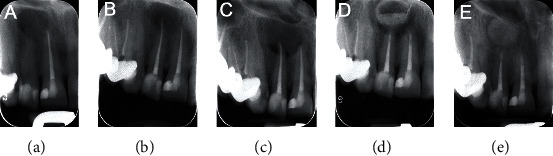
Periapical radiographs: (a) preoperative image of teeth #12, showing a large endodontic lesion; (b) immediate postendodontic radiograph; (c) at two-month follow-up, the patient returned with abscess formation and a larger periapical lesion; (d) applying bone substitute material during surgical retreatment; (e) at 6-month follow-up, bone healing can be observed.
